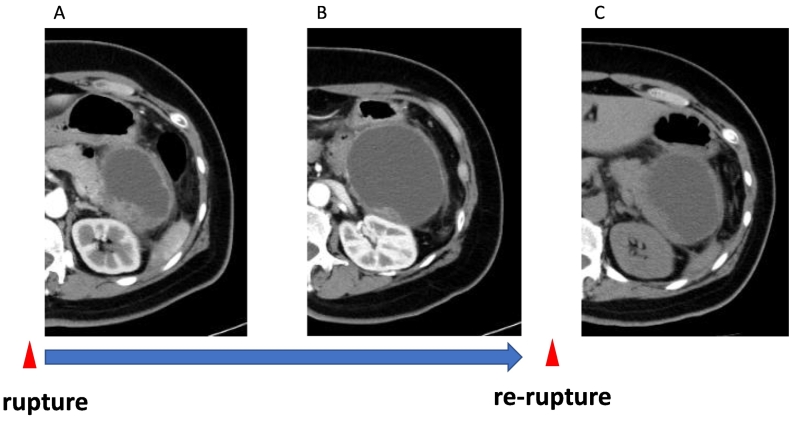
A; CT at first visit, B; one month after, C; after re-rupture. At first visit, the cyst was 80 mm in size and had solid conponents inside. One month after, the cystic lesion had gradually grown to 96 mm and solid components became slightly smaller. 30 days after the follow up CT, she presented with sudden abdominal pain. We diagnosed with re-rupture because of the shrinkage of the cystic lesion.
